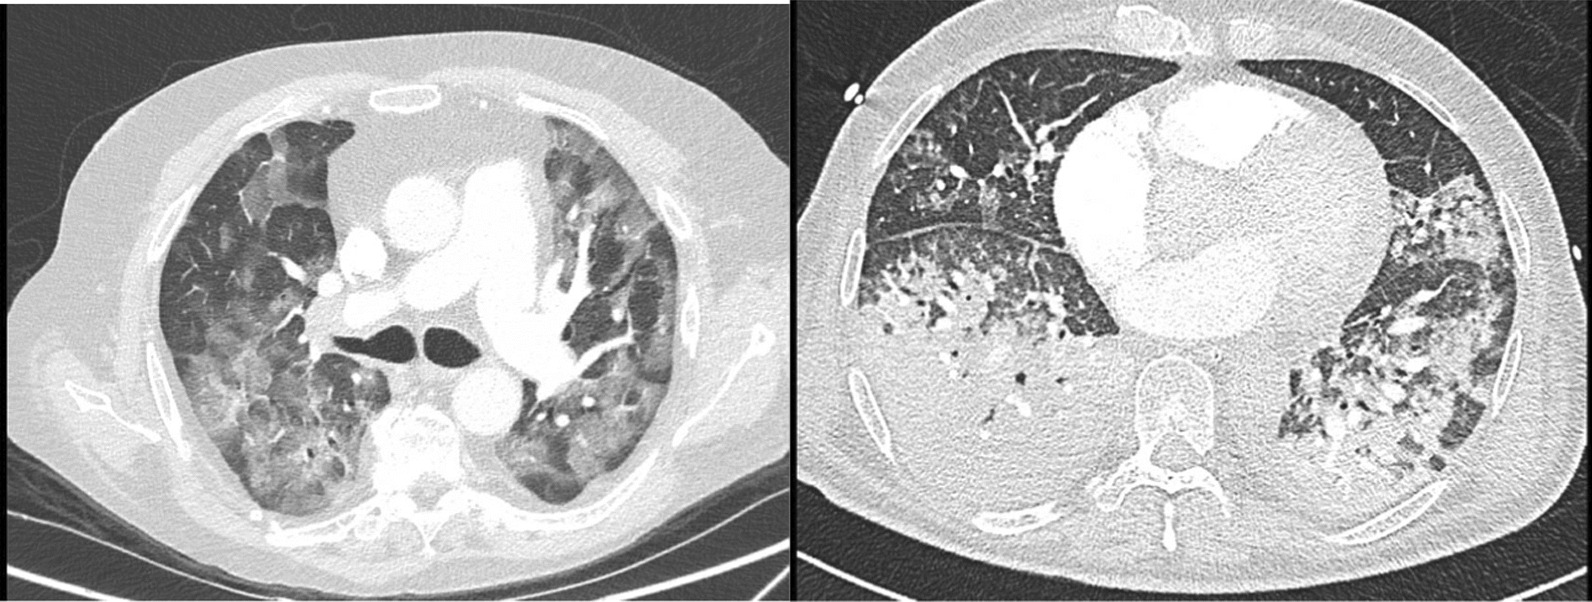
CT scan of two ARDS patients with different potential for lung recruitment. This figure shows images of two different patients, both fulfilling the criteria for ARDS. The CT scan in the left panel is characterized by extensive ground glass appearances, but limited consolidations, whereas the patient in the right panel has extensive gravity dependent consolidations. Obviously, the potential for lung recruitment with PEEP is much higher in the patients in the right panel
